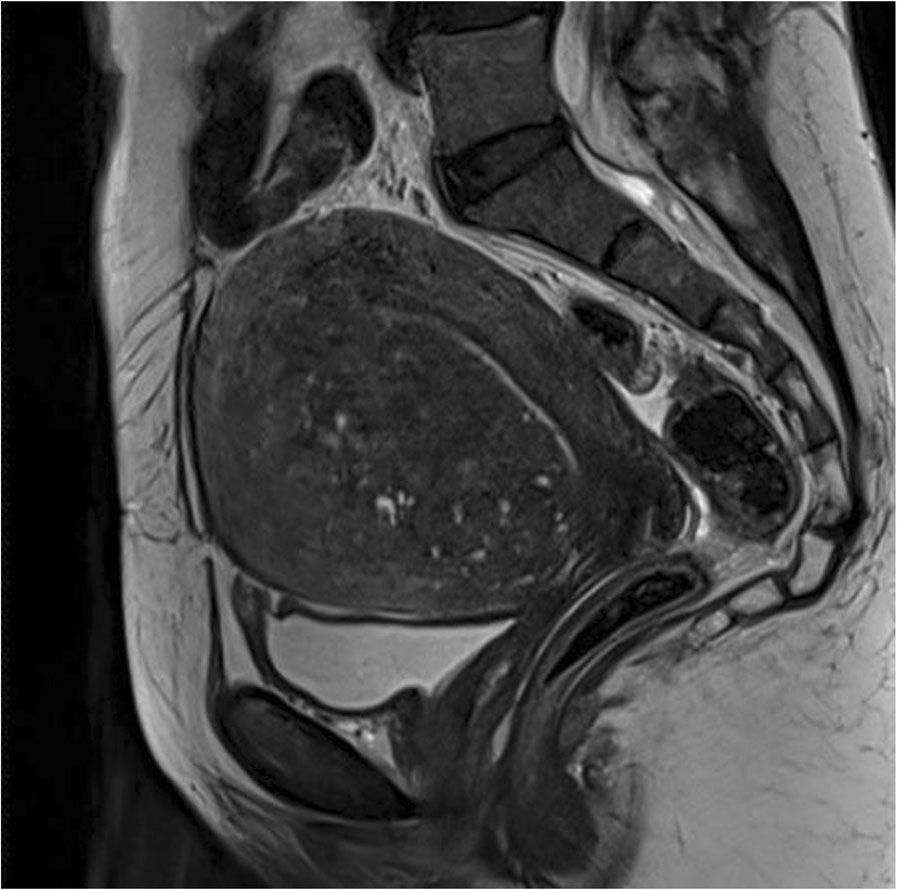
T2-weighted pelvic MRI revealed enlargement of the uterus, diffusely thickened junctional zone, the junctional zone and the muscle demarcation line blurring, suggesting adenomyosis
